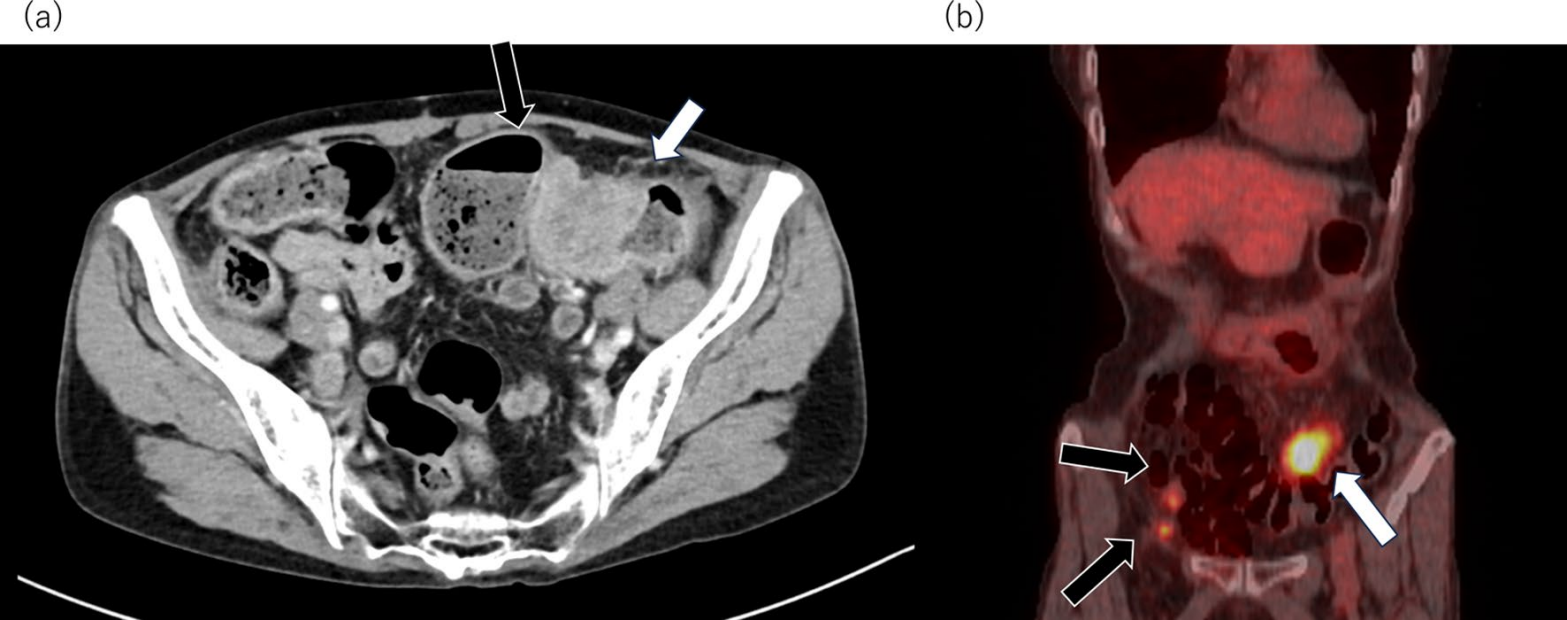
a Enhanced abdominal computed tomography showed irregularly enhanced wall thickening in the sigmoid colon (white arrow). The tumor was in contact with the transverse colon (black arrow). b Positron emission tomography (PET)–CT demonstrated accumulation in sigmoid colon tumor (SUVmax: 9.49, white arrow), and two peritoneal disseminations in the pelvis (SUVmax: 3.85, black arrow)
